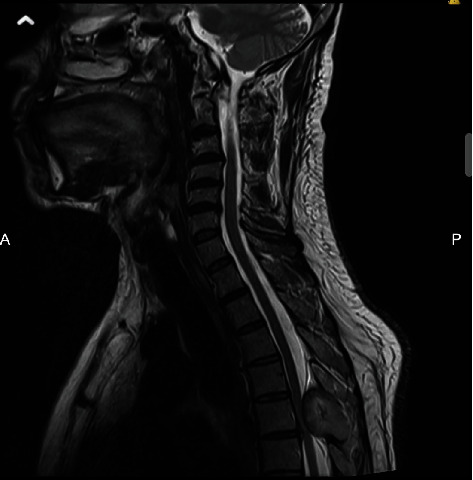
T2-weighted sagittal MR of the neck showing the spinal lesion at the level of T5.
